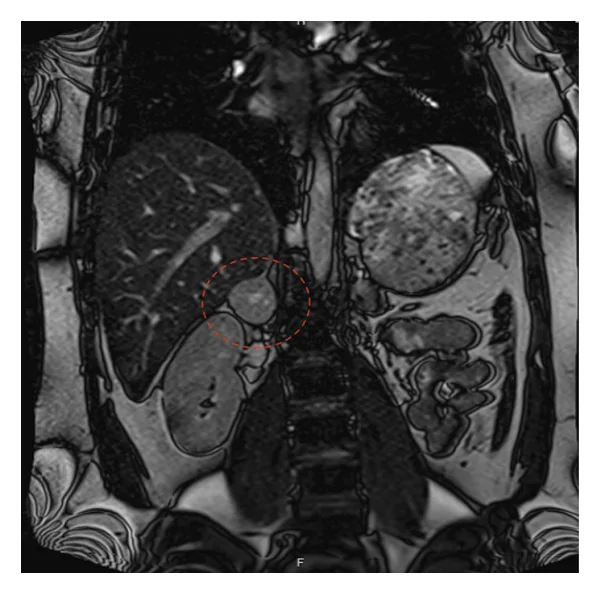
Coronal T2‐weighted magnetic resonance image of the abdomen and pelvis demonstrating a well‐circumscribed, rounded right adrenal mass (red dashed circle) with heterogeneous signal intensity, with central areas of necrosis and small foci of hemorrhage, consistent with likely pheochromocytoma. The left renal fossa is empty, confirming a solitary right kidney with normal corticomedullary differentiation. The liver and spleen appear unremarkable.
